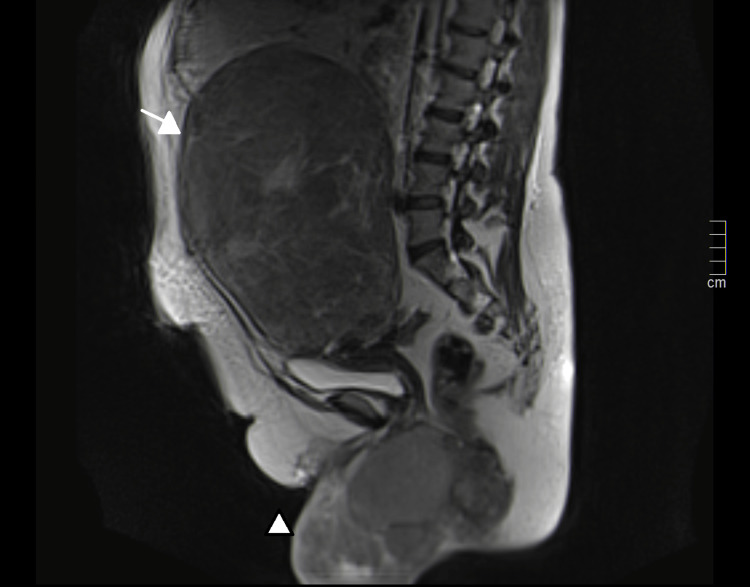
T2-weighted sagittal magnetic resonance imaging showing a hypointense uterine mass (arrow) and vulvar and perineal masses (arrowhead)
